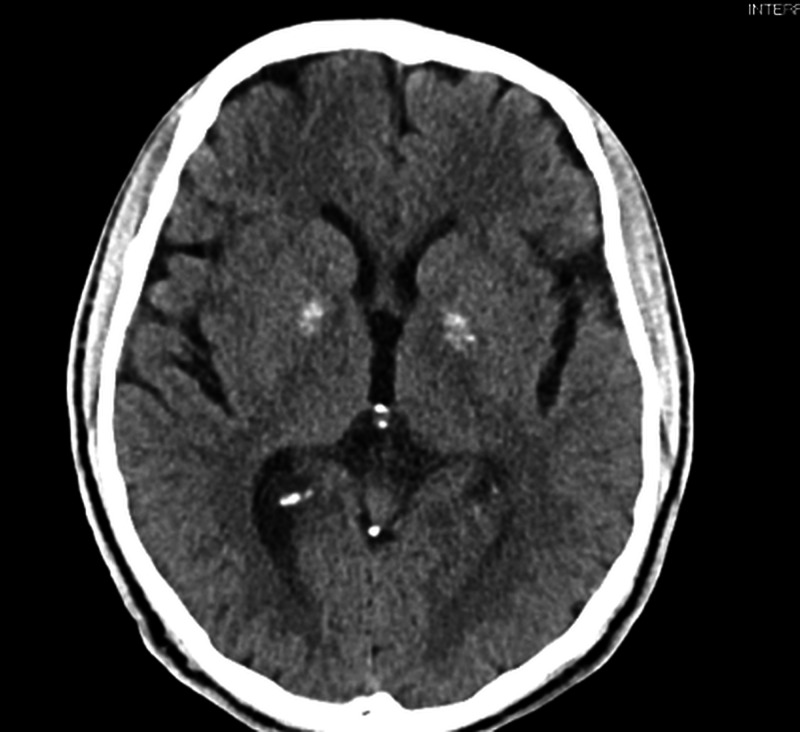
A transverse plane CT scan of the patient’s brain showing calcification limited to the anterior portion of the globus pallidus externus.
